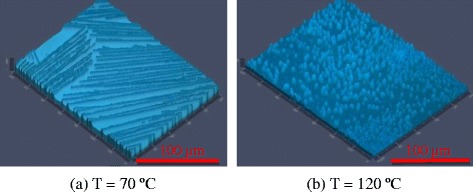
CLSM images of spray-on PbCl_2_ thin films made on ultrasonically vibrating substrates kept at a 70 °C and b 120 °C. The samples were annealed at the same temperature as the substrate temperature for 15 min. In (a), roughness = 159 nm and coverage = 46 %, and in (b), roughness = 55 nm and coverage = 68 %. The fabrication conditions of PbCl_2_ films are the same as those of runs (1–3) for (a) and runs (4–6) for (b), and TIS is irrelevant in this case. At 70 °C, PbCl_2_ film has a rode-like topography; therefore, the perovskite film made at this temperature is also rode-like, while at 120 °C, the PbCl_2_ film is grainy and so is the ensuing perovskite film (c.f. Fig. 4)
